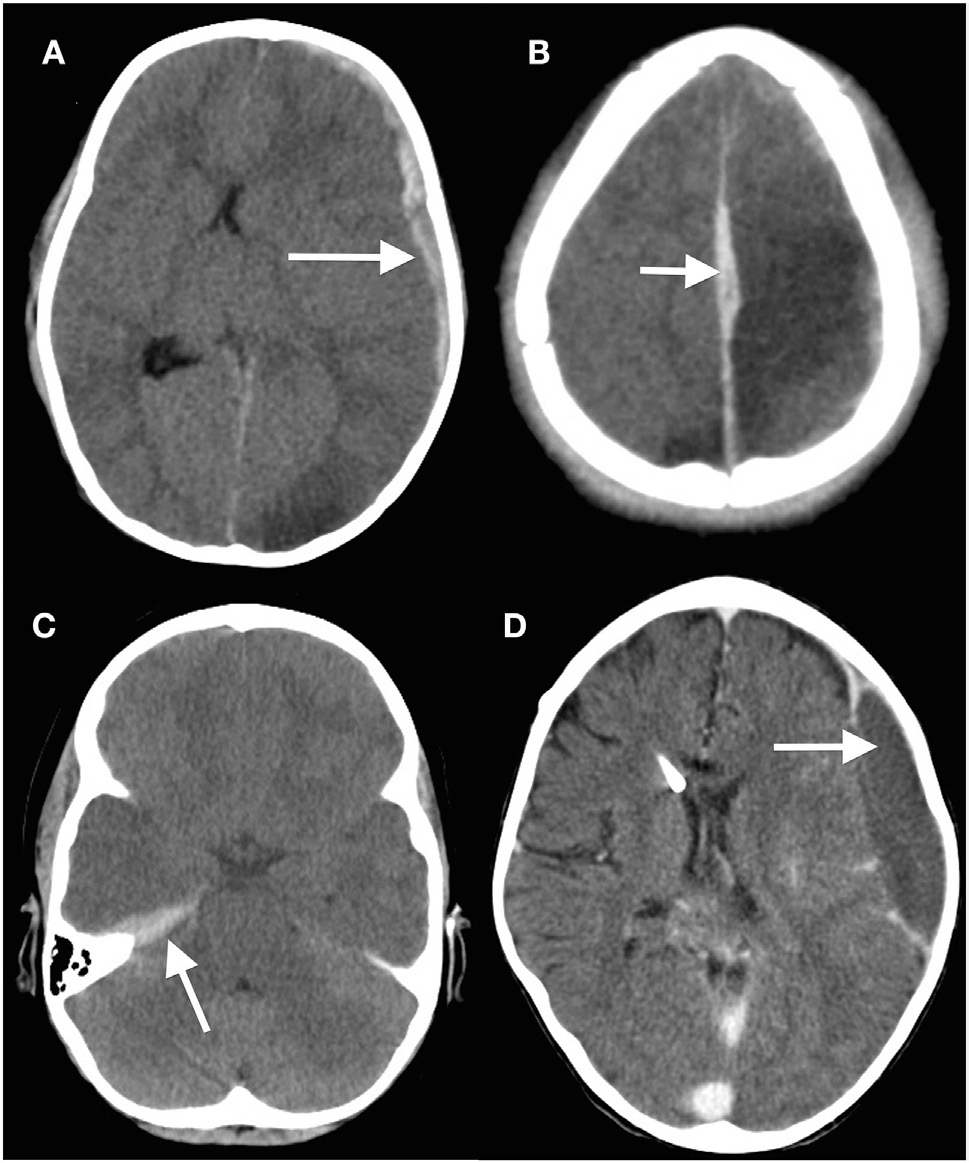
Subdural hematomas in children. (A) Head computed tomography (CT) scan showing a typical acute subdural hematoma with a hypodensity in the ipsilateral posterior cerebral artery territory; (B) interhemispheric subdural hematoma (arrowed) with adjacent venous hypodensity; (C) subtle subdural hematoma situated on the tentorium beneath the temporal lobe; (D) minor knocks to the head can easily cause a subdural hematoma (arrowed) in children with ventriculoperitoneal shunts, especially if there is a degree of overdrainage from the shunt (13) (modified).
